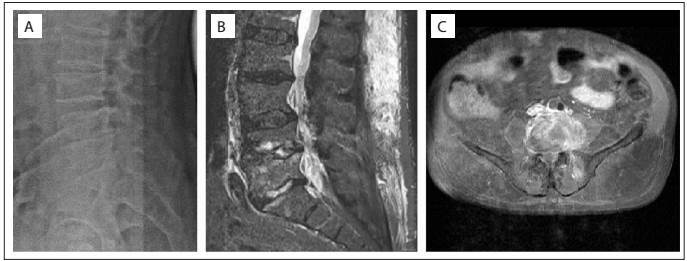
Radiography and magnetic resonance imaging (MRI) of lumbosacral spine. (A) Lateral radiograph showing irregular vertebral plates of L4 and L5, and reduction of disc spaces L4-L5 and L5-S1. (B) MRI in sagittal view with T1 fat-saturation contrast, showing spondylodiscitis of L4-L5, with subligamentous inflammatory tissue and reduced disc height of L4-L5 and L5-S1. (C) MRI in axial view with T1 gadolinium contrast, showing inflammatory tissue involving L4 and left psoas muscle.
